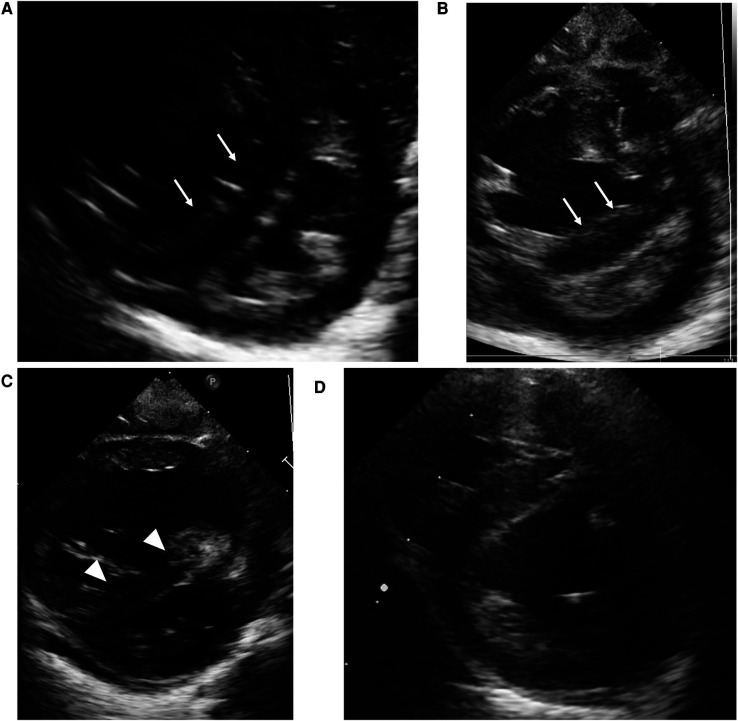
Case 1: echocardiography in the parasternal short axis view. Echocardiography at birth (A) and at the time of PH crisis (B) showing septal bowing to the left (white arrow) and a D-shaped left ventricle that indicates over-systemic pulmonary arterial pressure. On the day after the initiation of high-dose epoprostenol (C), left sided deviation of intraventricular septum (white arrowhead) ameliorated compared to at the time of PH crisis. At one year of age (D), the interventricular septal shift and left ventricle compression have improved.
